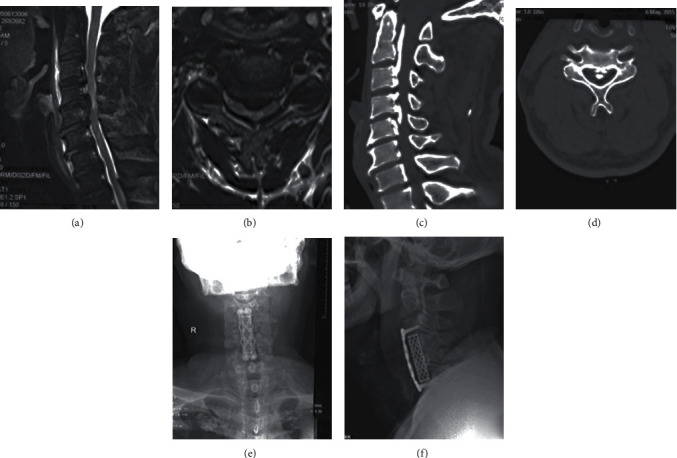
A 54-year-old male patient with SCIWORA associated with C-OPLL received anterior cervical decompression and fusion. (a) Intramedullary high signal intensity (SI) in preoperative sagittal T2-weighted MRI scan. (b) Compressed spinal cord on preoperative axial T2-weighted MRI scan. (c, d) Cervical ossification of the posterior longitudinal ligament on sagittal and axial CT scans. (e, f) Anterior cervical decompression and fusion at C3-6 segment on postoperative plain radiographs (anteroposterior and lateral).
